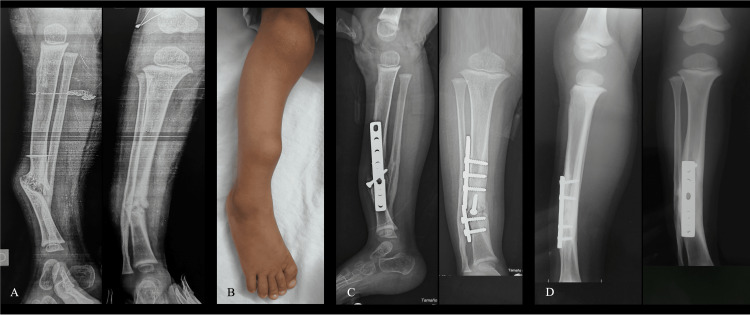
Nonunion of the distal tibia (Case 1) A and B: Radiographs and clinical aspects of a patient managed with a cast. Observe the hypertrophic nonunion with varus and antecurvatum deformity. C: The patient was submitted to open reduction and internal fixation with a plate, however, an implant failure occurred and the fracture did not heal. D and E: The patient was revised with new osteosynthesis with a plate. An autogenous bone graft was used. X-rays in the last follow-up show uneventful healing of the fracture with slight, acceptable residual varus.
